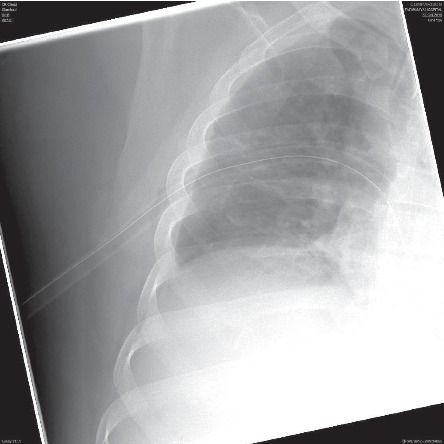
Intraoperative chest radiograph after insertion of intercostal tube showing normal lung expansion.
